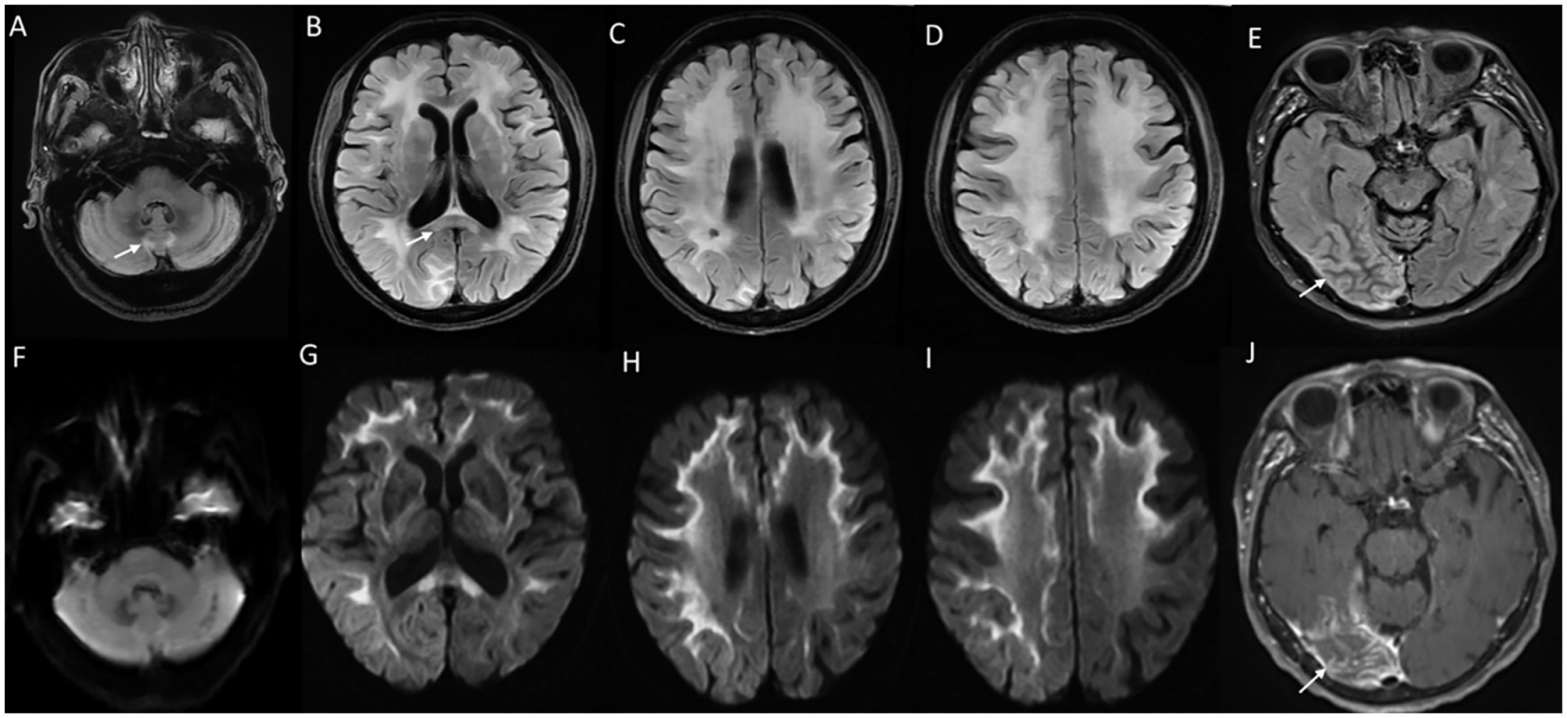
MRI brain findings in neuronal intranuclear inclusion disease (NIID). T2FLAIR sequences (A–D) reveal diffuse signal changes subcortical, periventricular, and deep white matter including splenium [arrow in (B)] and para-vermian region (A), along with corresponding DWI sequences (F–I) with high signal in a curvilinear pattern at the cortical gray–white matter junction of the supratentorial region. Imaging of a patient with NIID during recurrent encephalitis-like presentation revealing swelling, and signal changes involving right occipital gray matter in the T2FLAIR images (E) and abnormal leptomeningeal enhancement in the post-contrast sequence (J).
